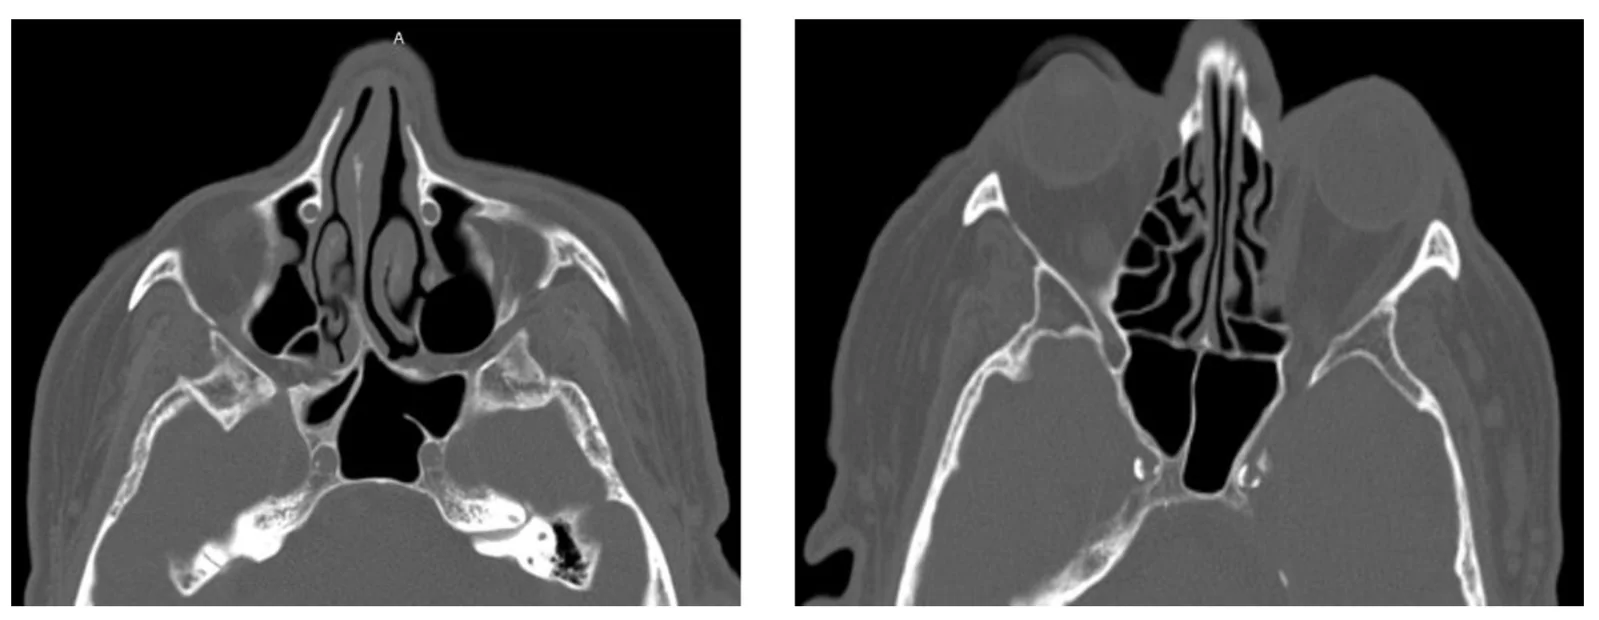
Sphenoid sinus bone CT dataset in axial planes.
